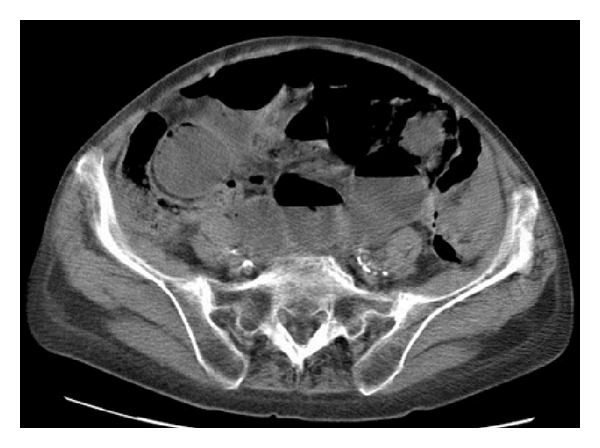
CT abdomen and pelvis showing moderately dilated small bowel loops and pneumatosis intestinalis.
